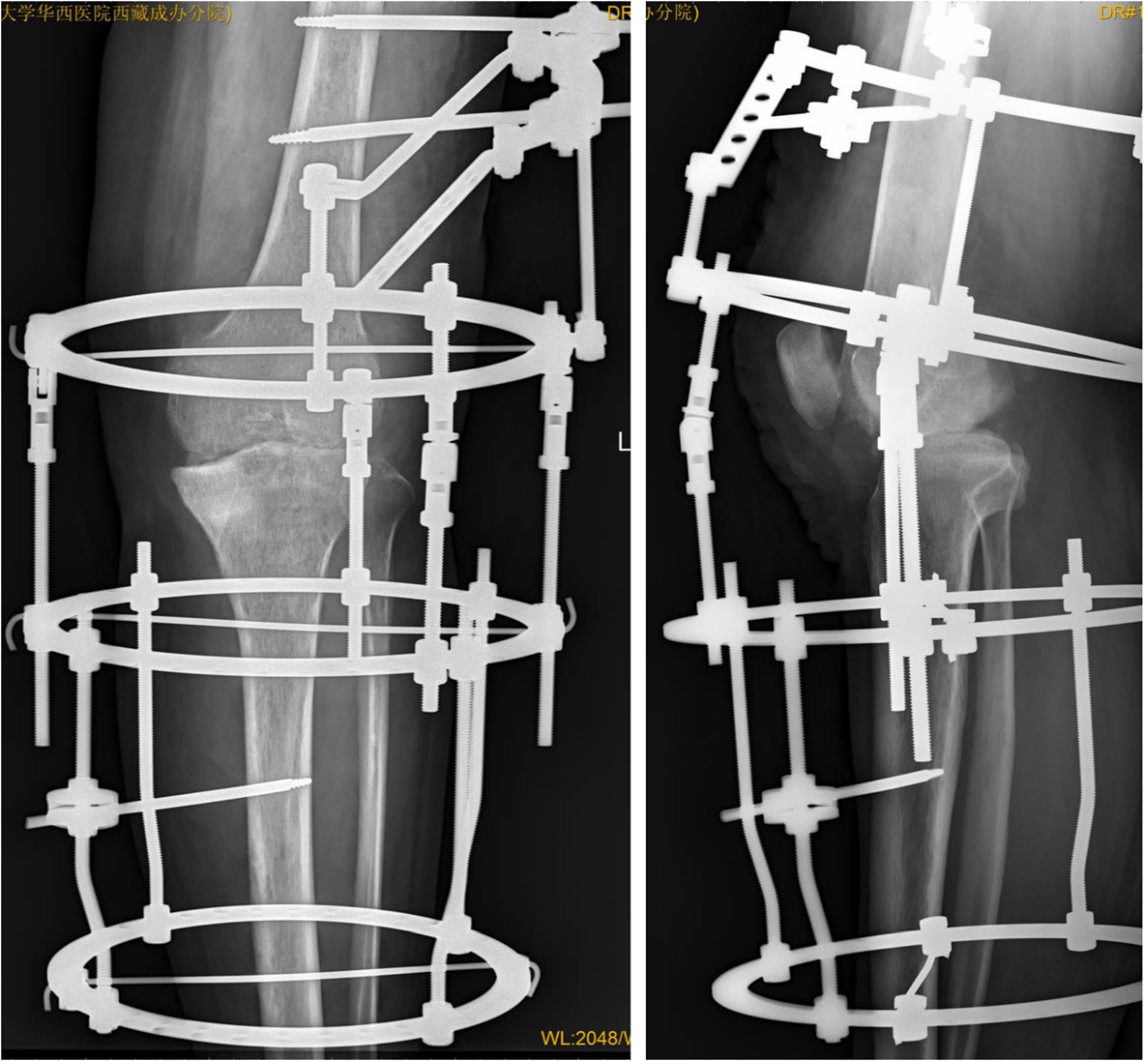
X-ray (anteroposterior and lateral): good bony contact after operation
